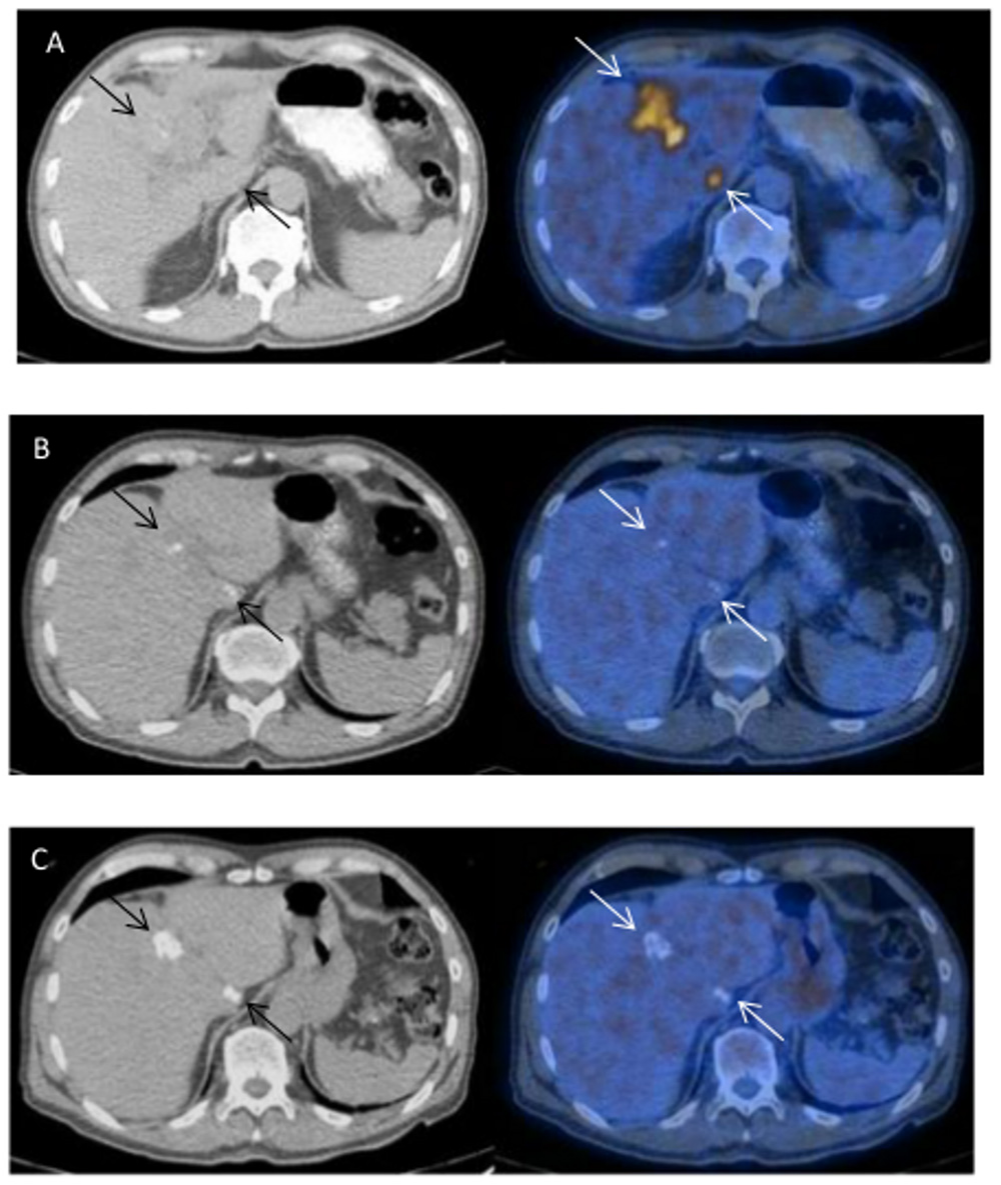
(A9): Baseline and follow-up FDG-PET/CT scans without i.v. contrast. a) Large and small AE-lesions (arrow) at the time of diagnosis (2003) with very strongly increased FDG uptake. b) The lesions became FDG-negative within two years of albendazole treatment. c) Progressive calcifications of AE lesions over the next 6 years after abrogation of chemotherapy (2011). No signs of FDG uptake are present.
